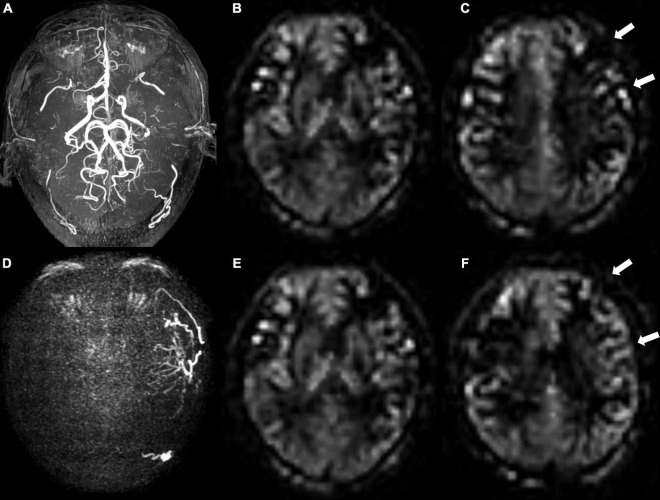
A 48-year-old male patient with moyamoya angiopathy. (A) Presurgical MR angiography image. (B,C) Two-slice ASL images of ASPECT regions, and preoperative collateral score was 10 in (B) and 9 in (C). (D) Postoperative super-selective 4D MRA images of external carotid artery. Postsurgical collaterals were defined as poor (1 score according to Matsushima standard). (E,F) Same postoperative two-slice ASL images as (B,C). Postoperative collateral scores increased (white arrows) to 10 in (E) and 11 in (F).
